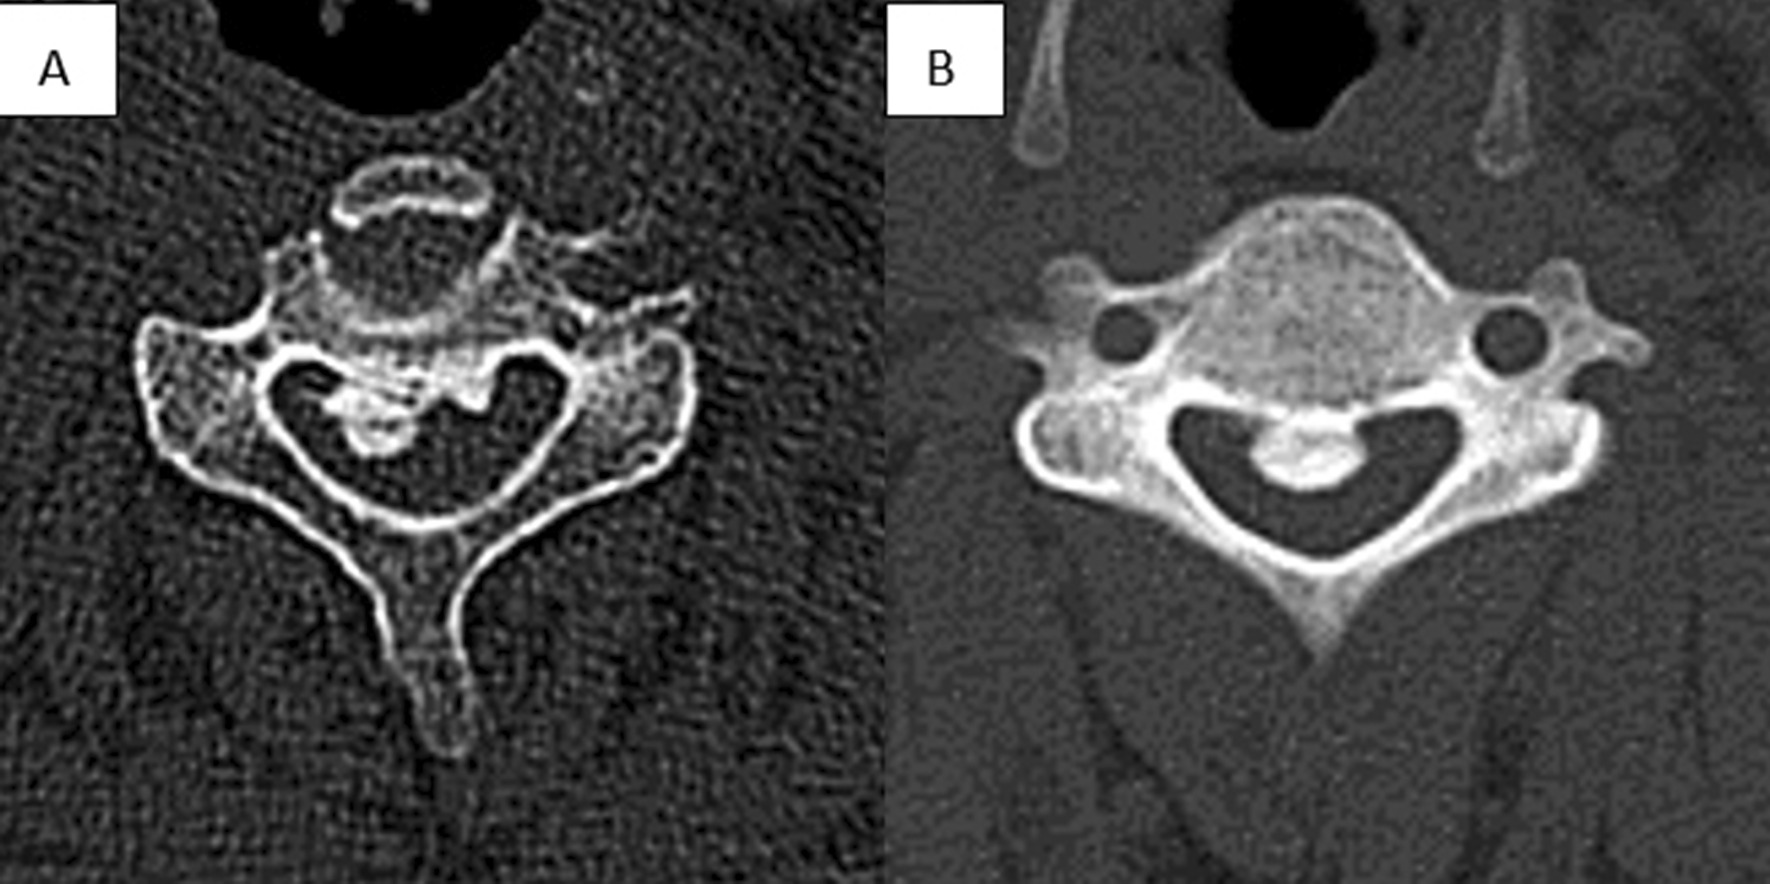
Axial ossified pattern of OPLL on CT. Central OPLL: the posterior prominence of the OPLL is located in the middle one-third of the spinal canal. A Lateral OPLL. B Central OPLL
